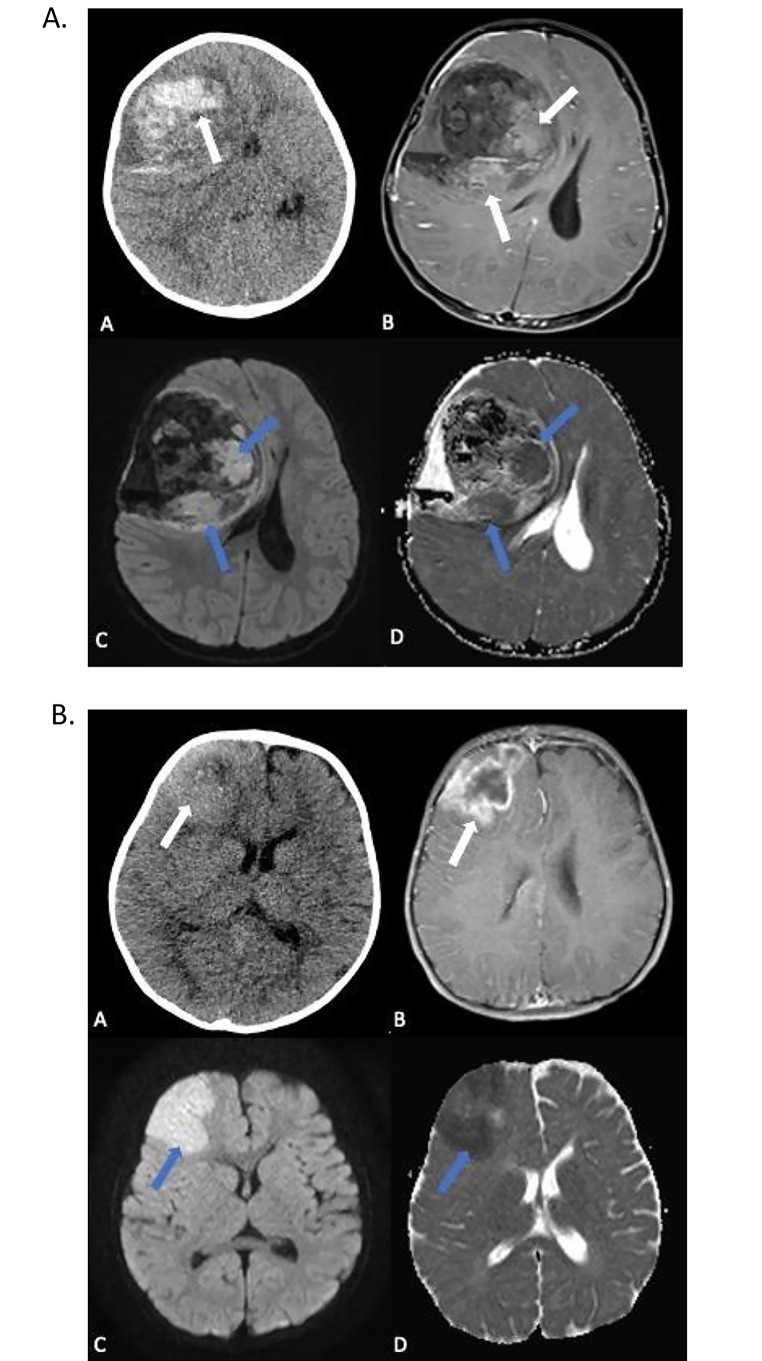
a: Initial imaging studies for patient 1. (A) Non-contrast axial CT scan of head shows a large hemorrhagic right frontal lobe mass lesion with mass effect and midline shift (white arrow). (B) Axial post-contrast T1-weighted MR image demonstrates right frontal heterogenous mass with enhancing soft tissue components (white arrows). (C) Diffusion weighted image and (D) ADC demonstrate restricted diffusion in the areas of enhancing soft tissue components, appearing bright on diffusion and dark on ADC (blue arrows) b. Initial imaging studies for patient 2. (A) Axial non-contrast CT scan of the head shows an ill-defined hyperdense mass with punctate calcifications and a small necrotic area (white arrow). (B) Postcontrast T1-weighted MR image demonstrates heterogeneously enhancing frontal lobe mass with necrotic area (white arrow). (C) DWI and (D) ADC images show restricted diffusion indicating highly cellular components (blue arrows)
